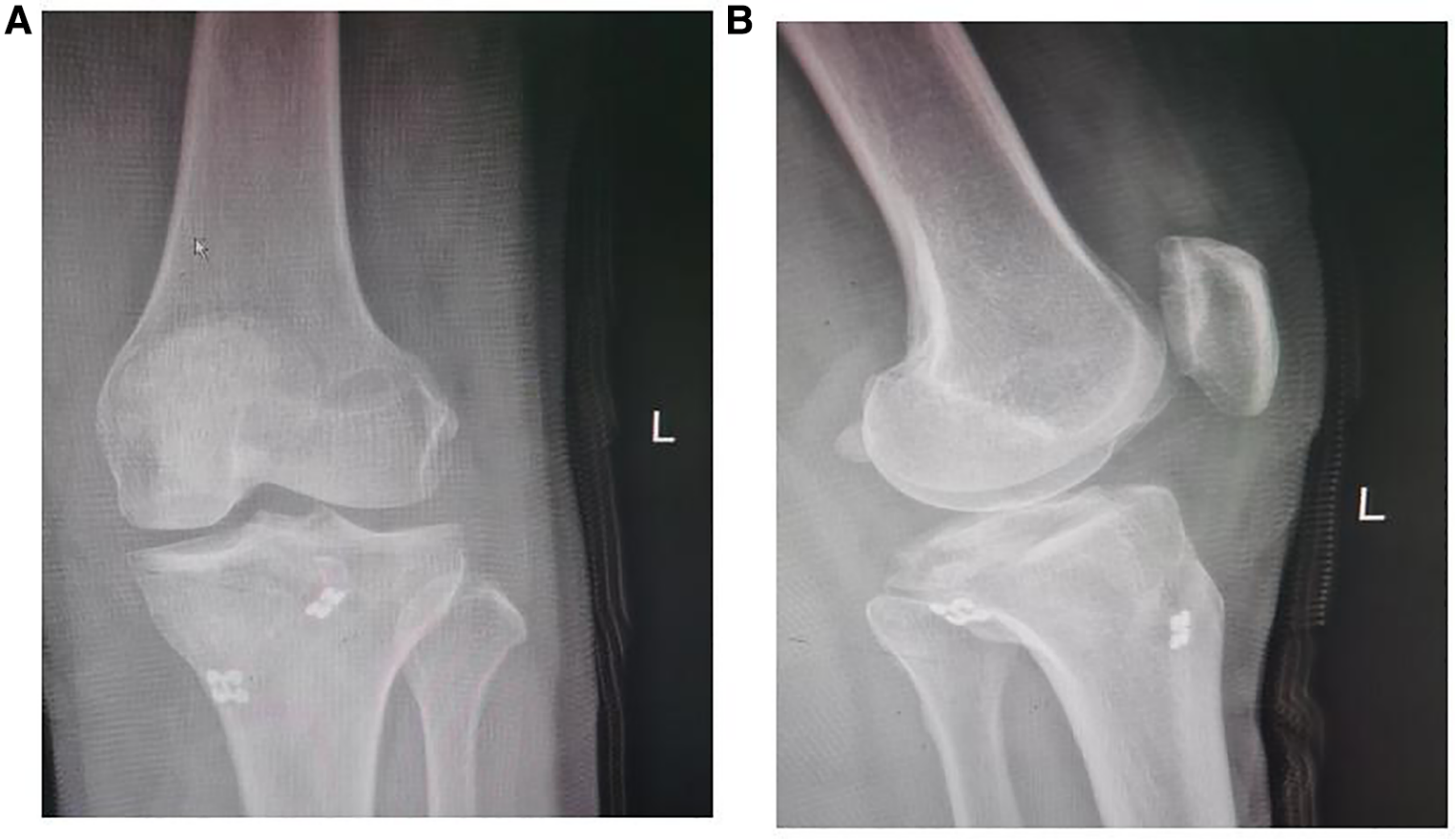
Postoperative radiograph of double button plate fixation (20 months) cannulated screw fixation. Follow-up x-rays showed that the avulsion fracture fragments of the tibial attachment point of the PCL healed well, and there was displacement or implant breakage of the fracture fragments.
